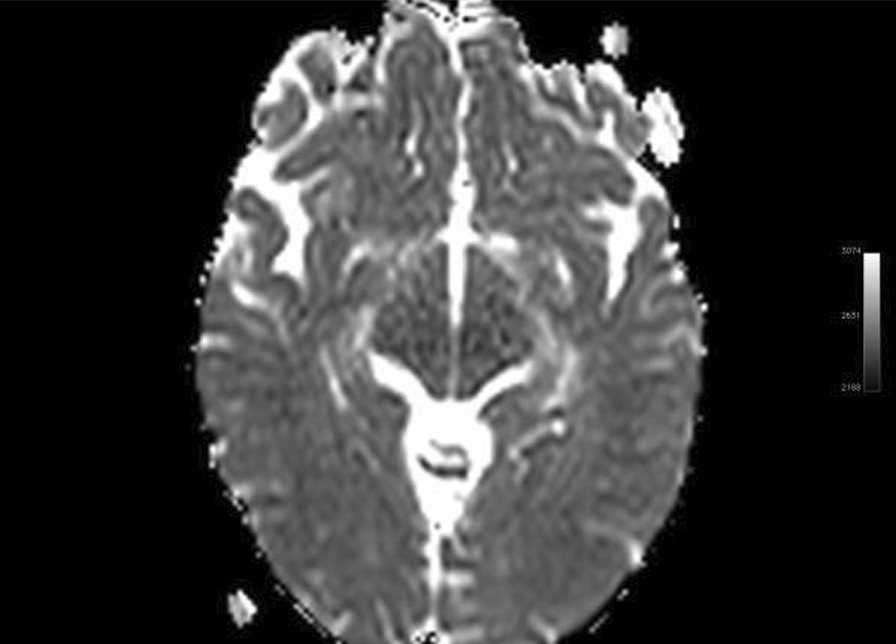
Axial ADC demonstrates T2 shine-through characteristics with high signal in the corticospinal tracts noted on the DWI with isotense signal on the apparent diffusion co-efficient map
